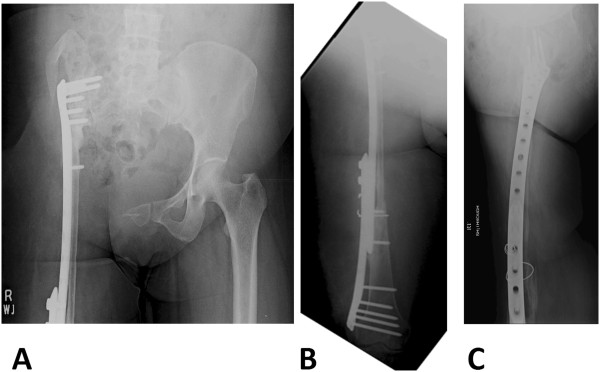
One year follow-up x-rays of pelvis (Figure 13
